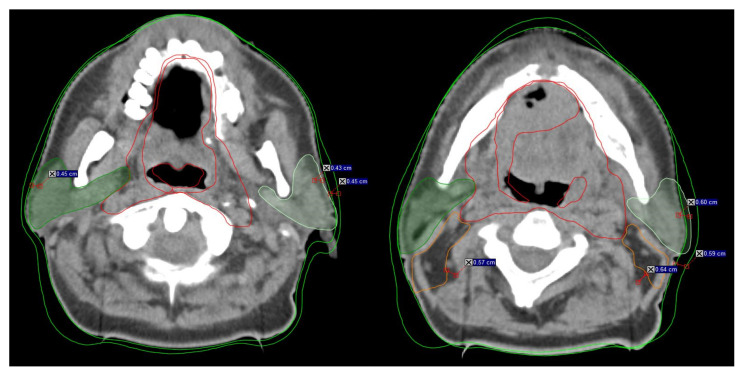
Example of the rapidly changing anatomy during head neck radiotherapy from treatment initiation to replanning. Visible changes >3 mm in the anatomy during RT indicate inclusion for B-ART. The distance mark on the scans represents the anatomy changes between initial CT (iCT) and replanning (rCT). Dark green indicates the right parotid gland, and light green indicates the left parotid gland. Red indicates CTV1, orange indicated CTV2, and green indicates the external contour (B-body).
